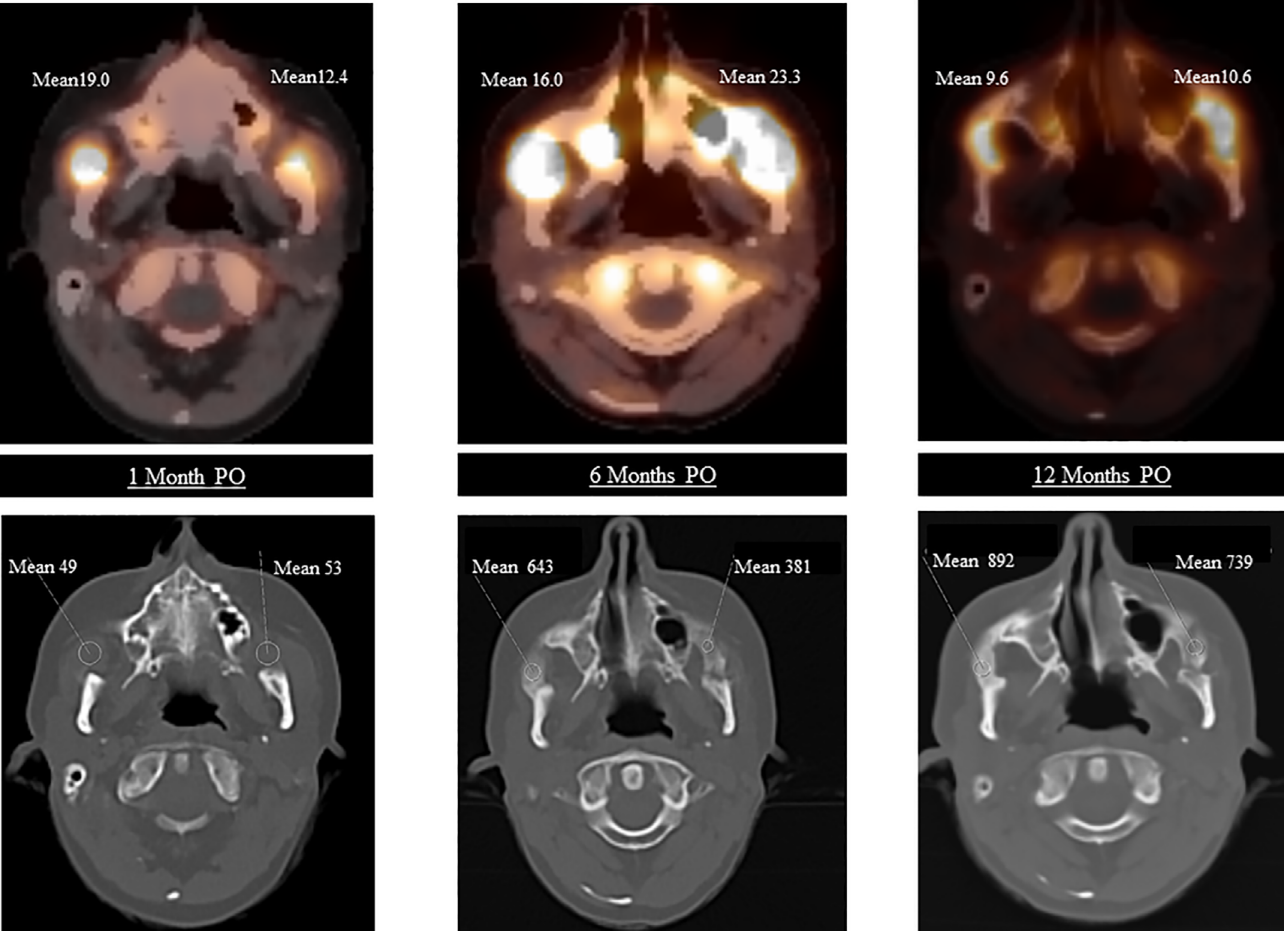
Axial [^18^F]‐NaF PET/CT images of the skull at (*A*) 1 month, (*B*) 6 months, and (*C*) 12 months PO. Upper panels show increased uptake of [^18^F]‐NaF as indicated by means of SUV. Lower panels show possibly HO after 1 month, increasing HO at 6 and 12 months as indicated by means in HU. PO = postoperatively; SUV = standardized uptake value; HO = heterotopic ossification; HU = Hounsfield units.
